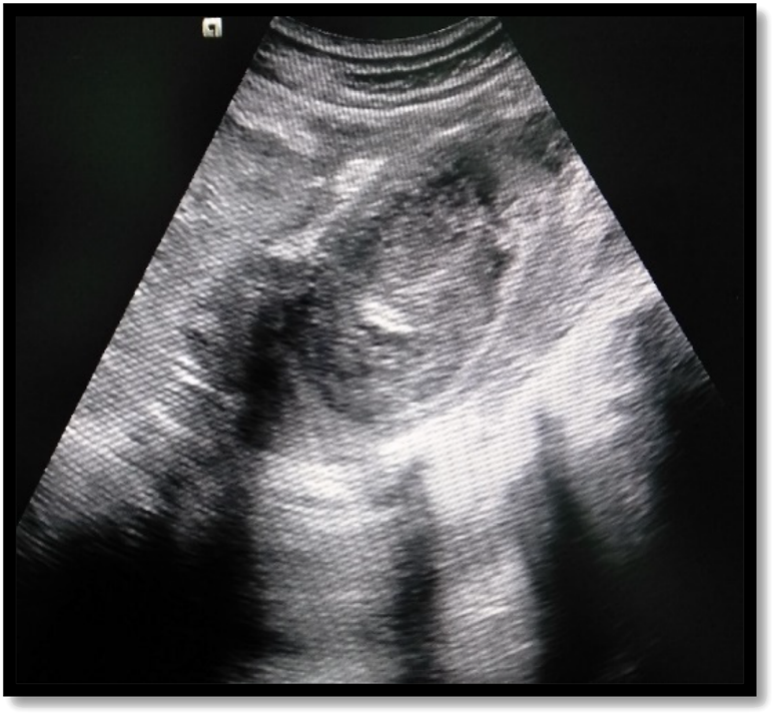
Ultrasonographic image shows heteroechoic collection in the psoas muscle.
